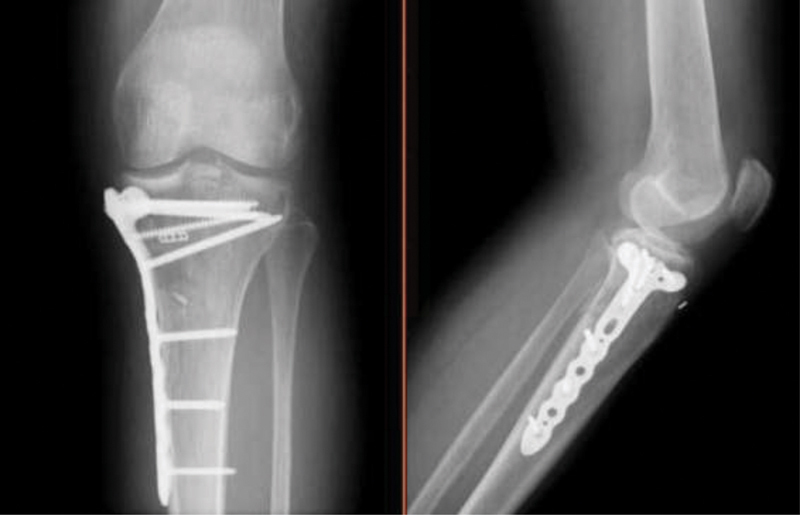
Anteroposterior and lateral radiograph at the 3-month follow-up.
